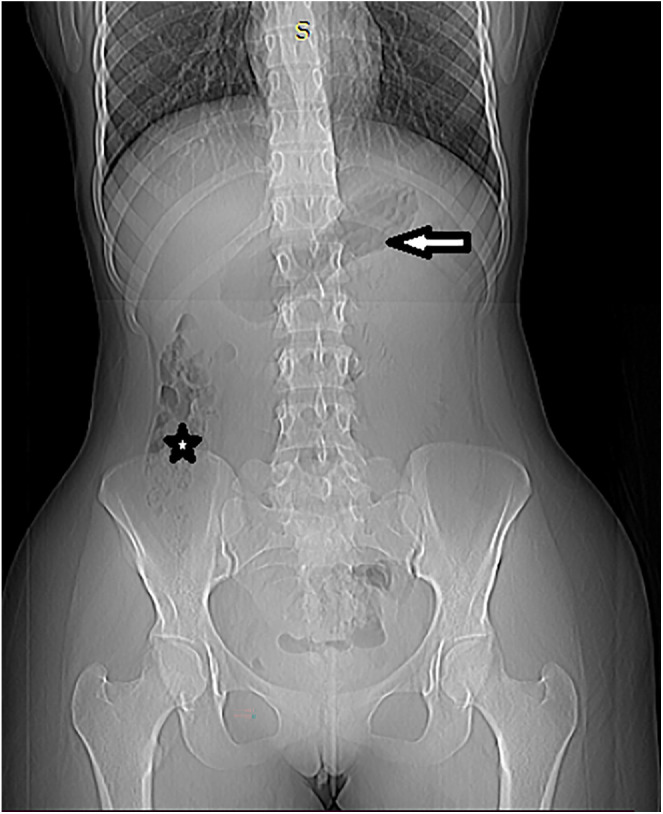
Plain abdominal radiography showing dilated stomach (arrow) and duodenum (star).
